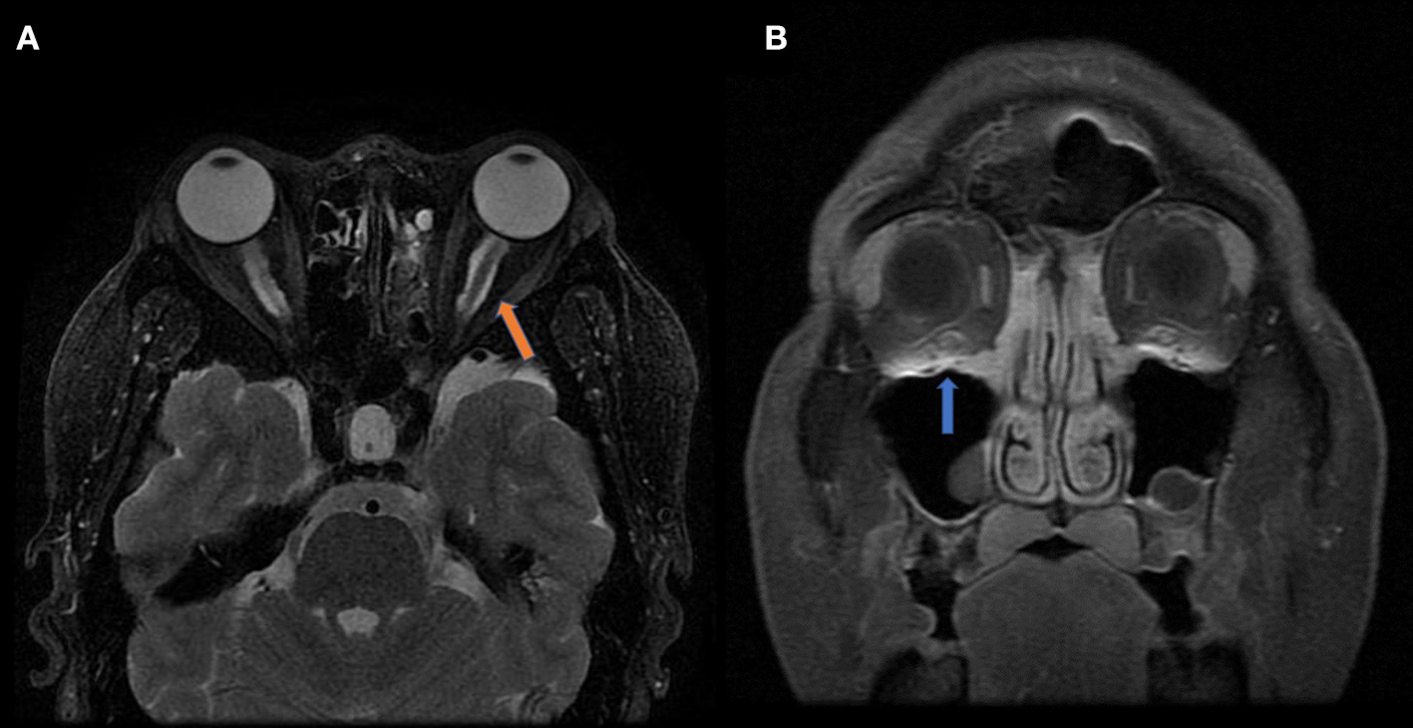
MRI findings of OPN patients with IgG-4 RD. (A) Coronal T2-weighted MRI showing swelling and high signal of the bilateral optic nerve sheath (arrow). (B) Coronal contrast enhanced T1-weighted MRI showing bilateral infraorbital nerve enlargement (arrow).
